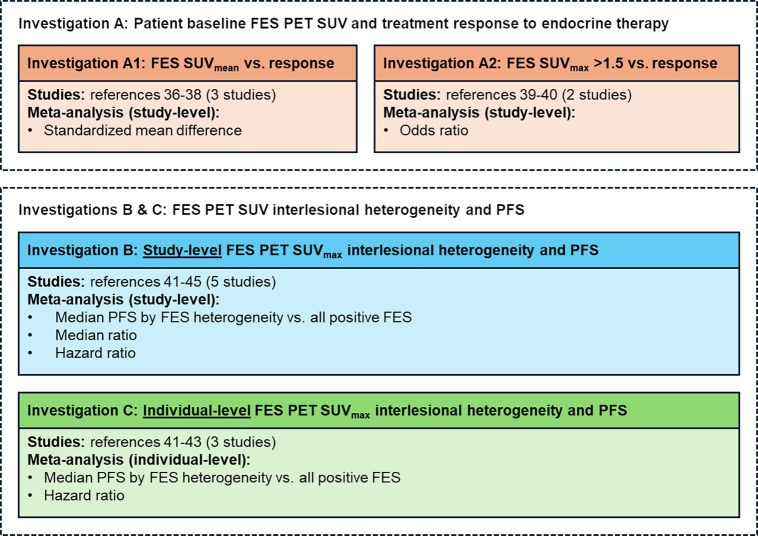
Investigations on relationships between [^18^F]FES PET SUV and response to ET or PFS.
